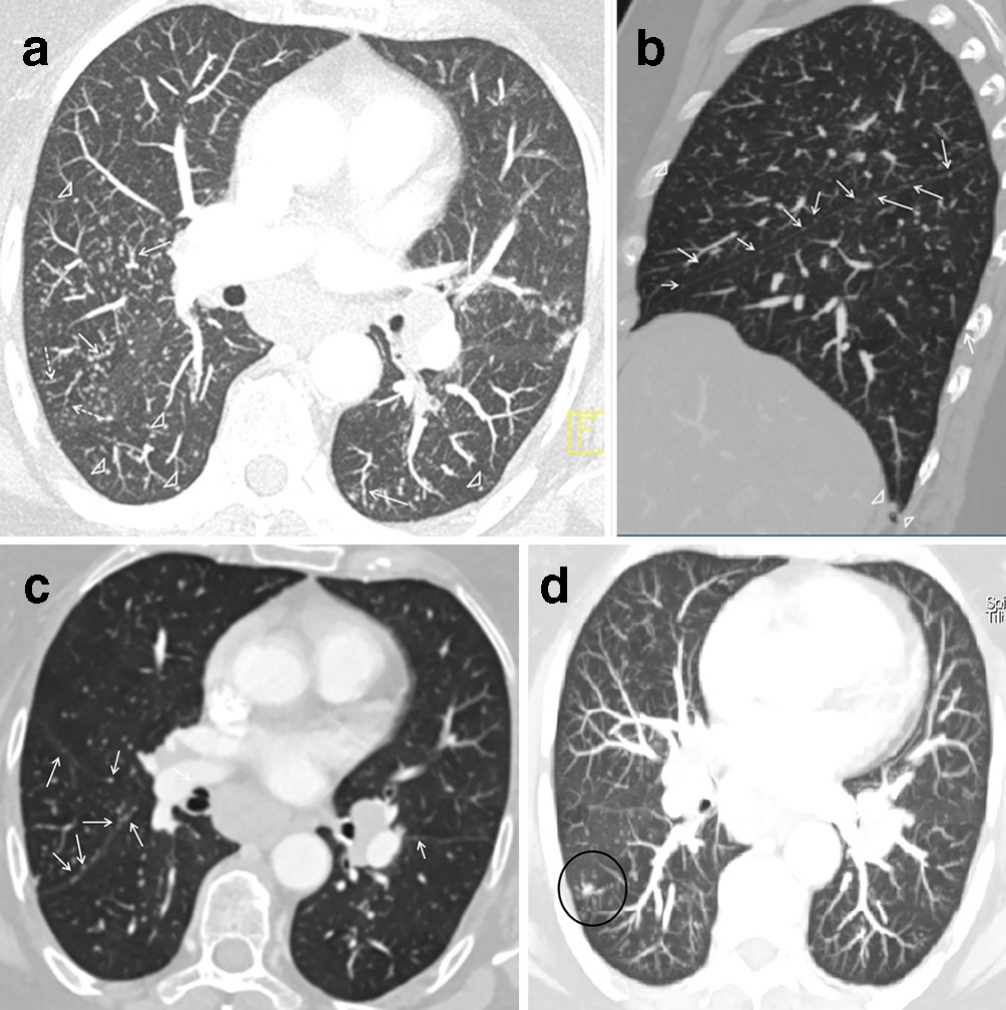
Contrast-enhanced CT scan of the thorax shows unnumbered small lung
